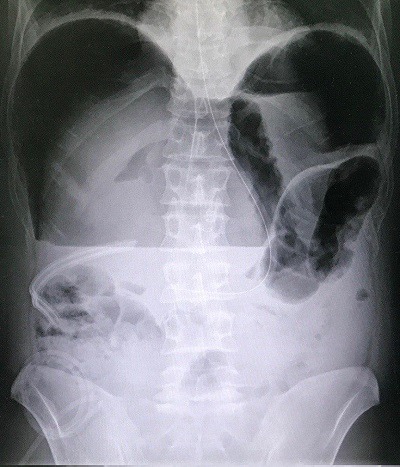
Second X-ray was 2 days after the first operation i.e. omental Graham patch operation, which showed a significant huge hydro pneumoperitoneum
